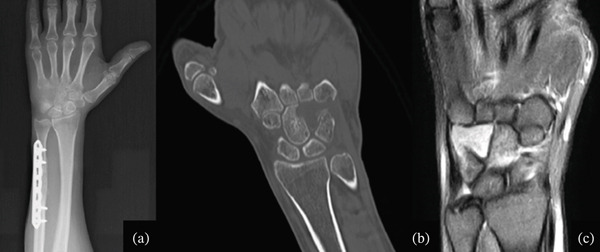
(a) Preoperative anteroposterior radiograph showing a destructive hamate lesion. (b) Computed tomography scan reveals cortical destruction of the hamate and minimal involvement of the capitate. (c) T2‐weighted STIR coronal magnetic resonance image demonstrating a hyperintense signal within the lesion.
